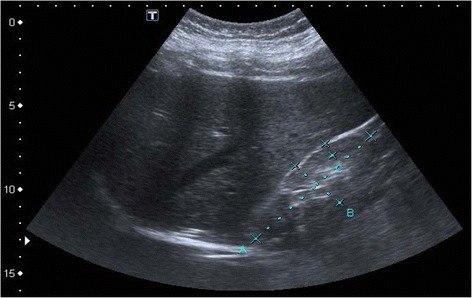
Abdominal ultrasonography of index patient. Right kidney is overall diminished in size (a-b: 88 × 33 mm, c: renal parenchyma 8 mm) and appears hyperechogenic compared to liver parenchym
